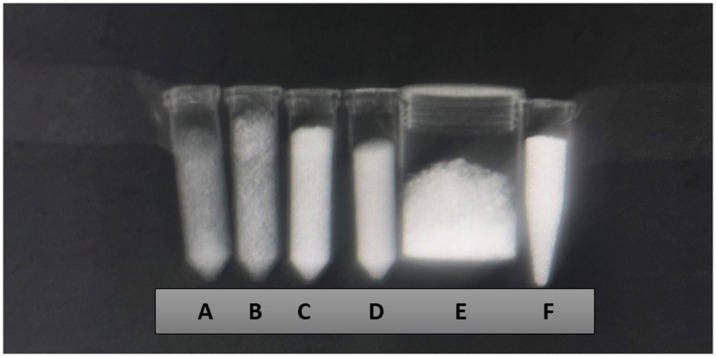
X-ray imaging using the wrist technique (mAs 20, ms 71, and 46 Kv) of the samples Zn-HA 10% (A), Sr-HA 10% (B), HA 01.256 CTT 1000 °C (C), HA microspheres (D), AuNP (E), and Cu-GO (F).
